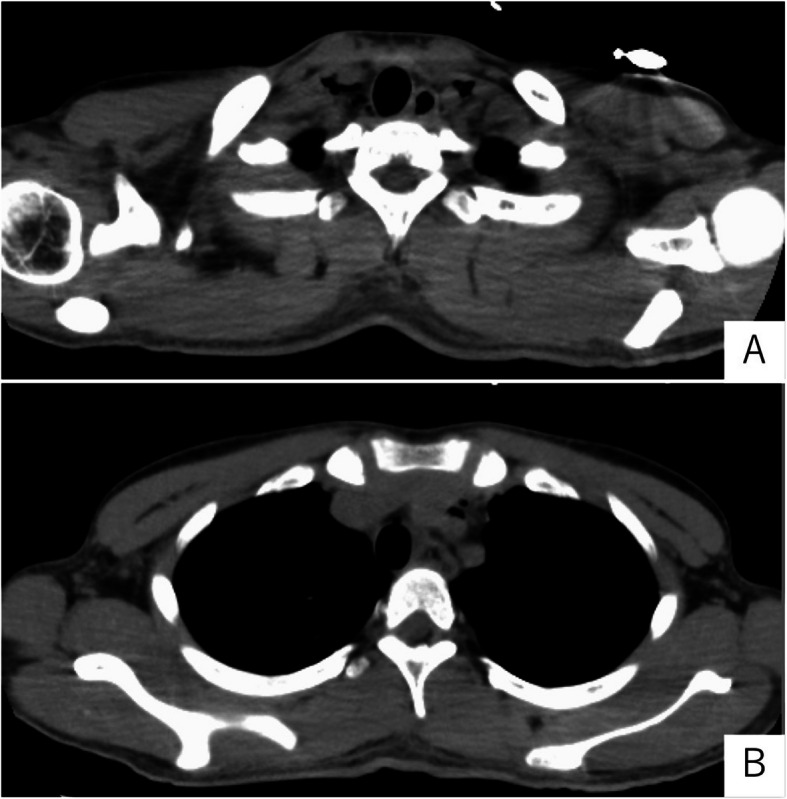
Follow-up computed tomography performed on hospital day 3
